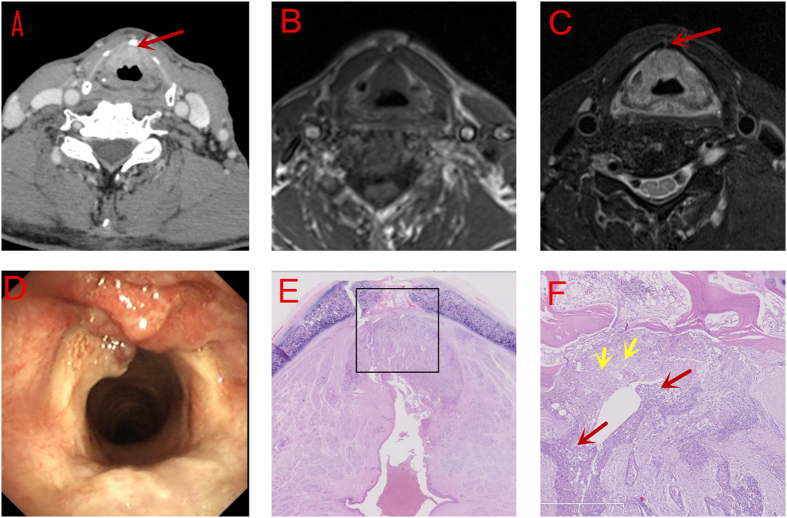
Involvement of Thyroid Cartilage without Invading into the Paraglottic Space. (A) CT scan: without involvement of thyroid cartilage (red arrow) (B) (T1WI)and (C) (T2WI, fat-suppression) MRI scan: Involvement of thyroid cartilage (red arrow) (D). Electronic laryngoscopic examination. (E,F) (amplified image within the rectangle in (E), 200×) Serial section: mild invasion of the thyroid cartilage (Red arrow: tumor cells; Yellow arrow: the impaired thyroid cartilage).
